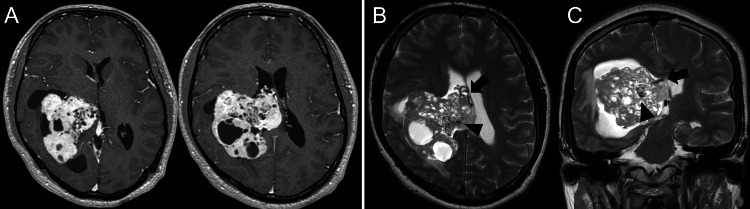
Preoperative MRI showing large hypervascular intraventricular tumor Contrast-enhanced T1-weighted axial image showing an enhancing multicystic tumor in the right lateral ventricle (A). T2-weighted axial and coronal images showing dilated intratumoral veins (black arrowheads) and large drainage veins running into the deep drainage system (black arrows) (B, C) MRI: magnetic resonance imaging
